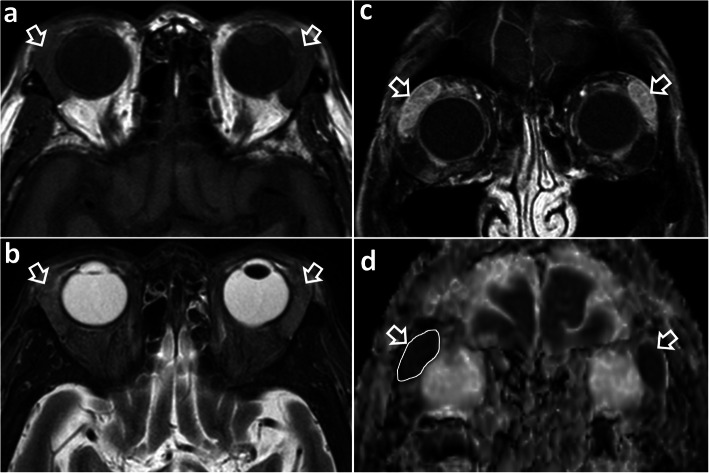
Magnetic resonance imaging of an 87-year-old woman with insidious onset of bilateral eyelid swelling and proptosis for 2 years, who had bilateral lacrimal gland MALToma. a Axial T1-weighted image shows bilateral enlargement of the lacrimal glands with homogeneous intermediate signal intensity (open arrows). b Axial T2-weighted image with fat saturation shows bilateral enlargement of the lacrimal glands with homogeneous intermediate signal intensity (open arrows). c Coronal T1-weighted post-contrast image with fat saturation shows bilateral enlargement of the lacrimal glands (open arrows) with moderate homogeneous contrast enhancement. d Coronal apparent diffusion coefficient (ADC) map shows bilateral enlargement of the lacrimal glands with markedly restricted diffusion (open arrows) with a very low ADC value of 0.56 × 10^− 3^ mm^2^/s (white contour)
